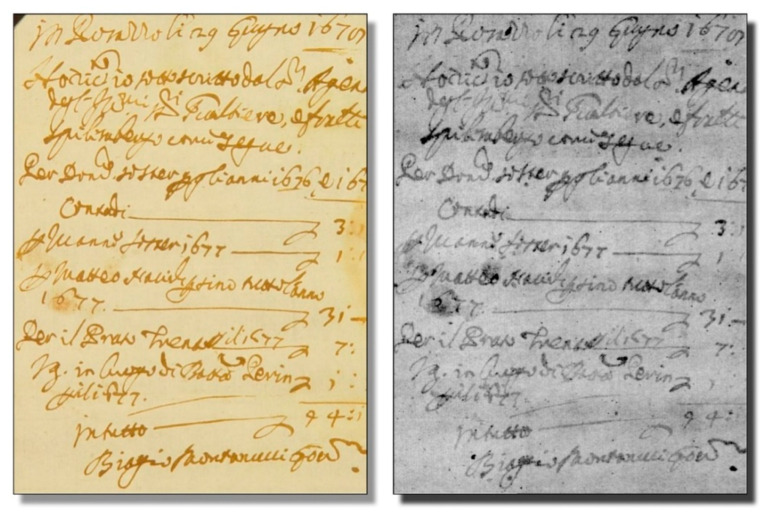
A manuscript revealed by radiography (right), compared to the corresponding visible-light picture [29,30,31].
